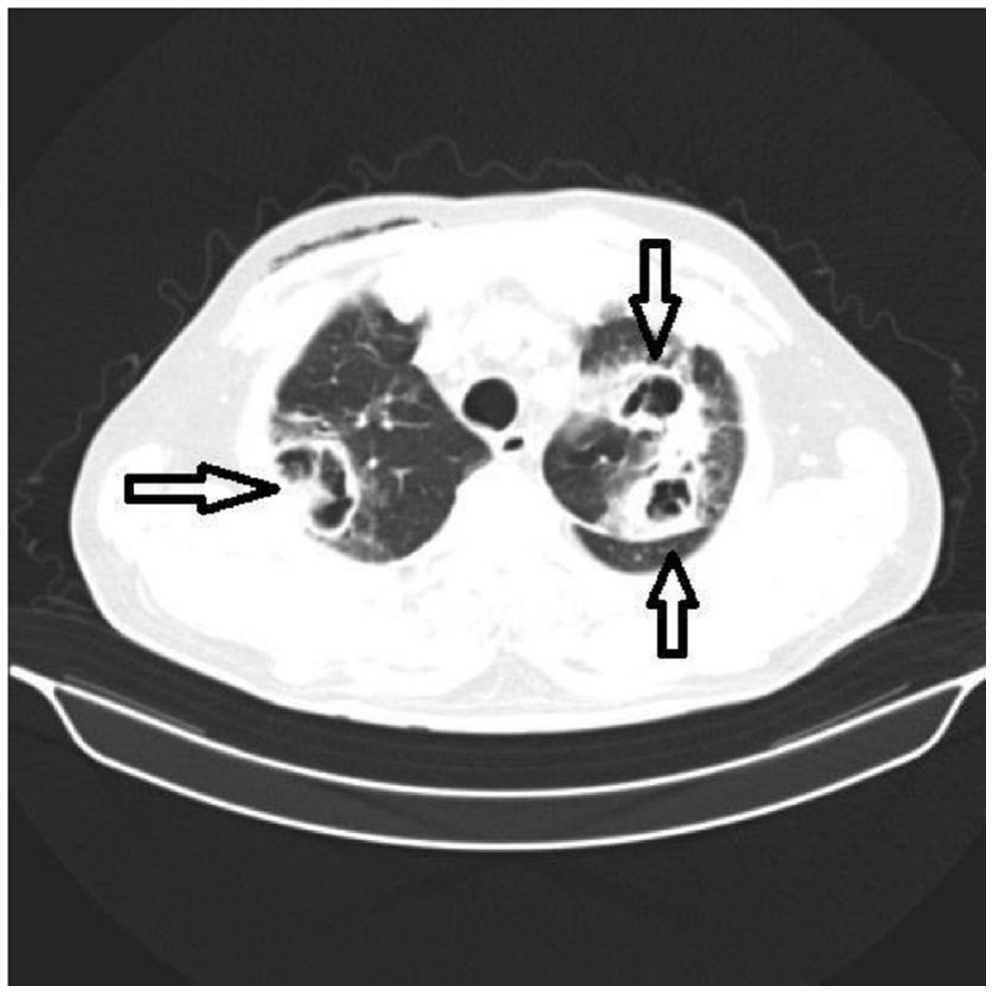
The CT scan shows cavitary lesions.
